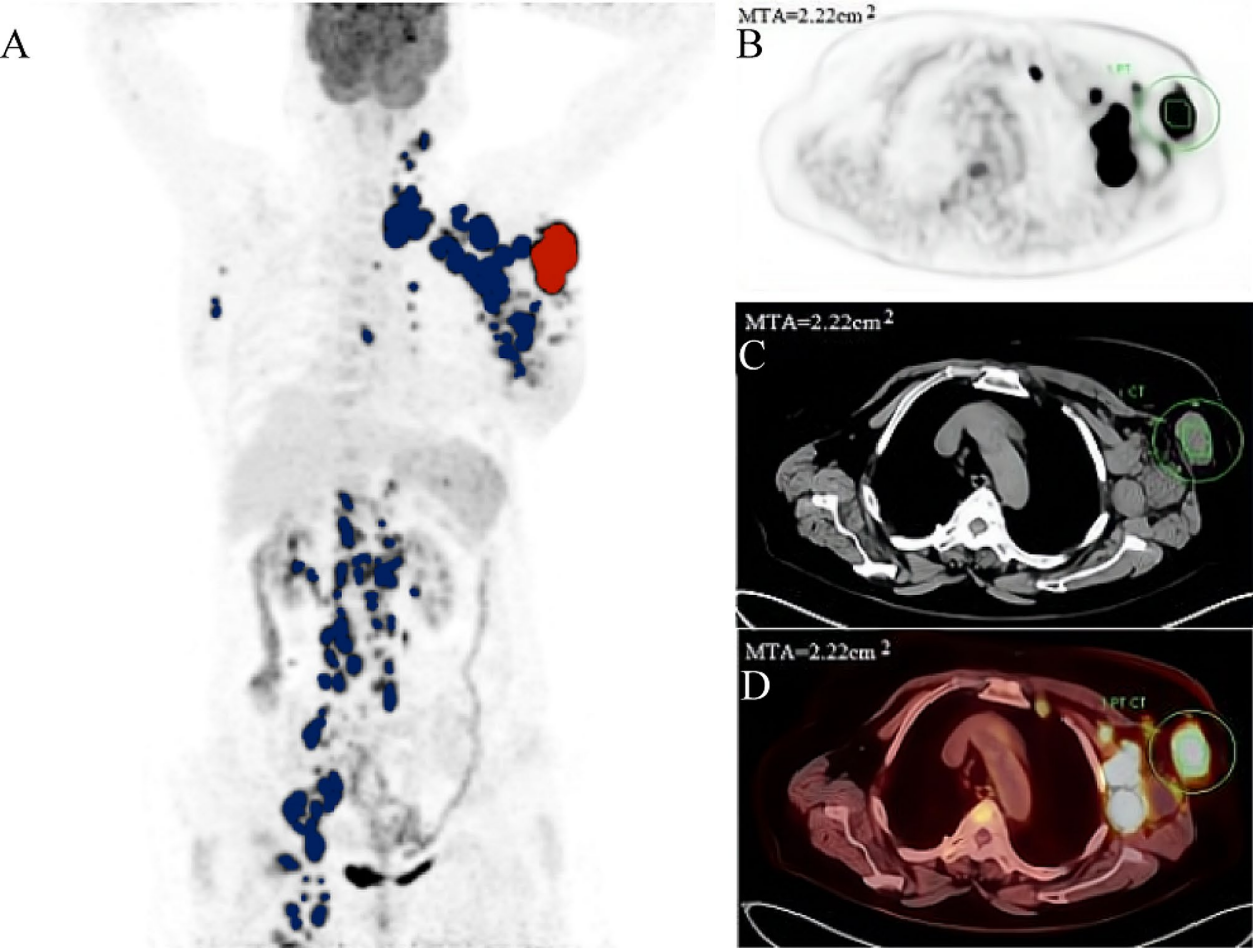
Delineation and calculation of the MTA based on ^18^F-FDG PET/CT images. A: Maximum intensity projection (MIP) is used to identify the largest lesion. All involved lesions are shown in blue, and the largest lesions used to obtain MTA are represented in red. B: PET tomographic reconstruction of cross-sectional images. C: CT tomographic reconstruction of cross-sectional images. D: The maximum cross-sectional area of the largest lesion on the ^18^F-FDG PET/CT tomographic fusion images to obtain the MTA, which can be automatically displayed in the TrueD system and delineated by a solid yellow line, with an MTA of 2.22 cm^2^
